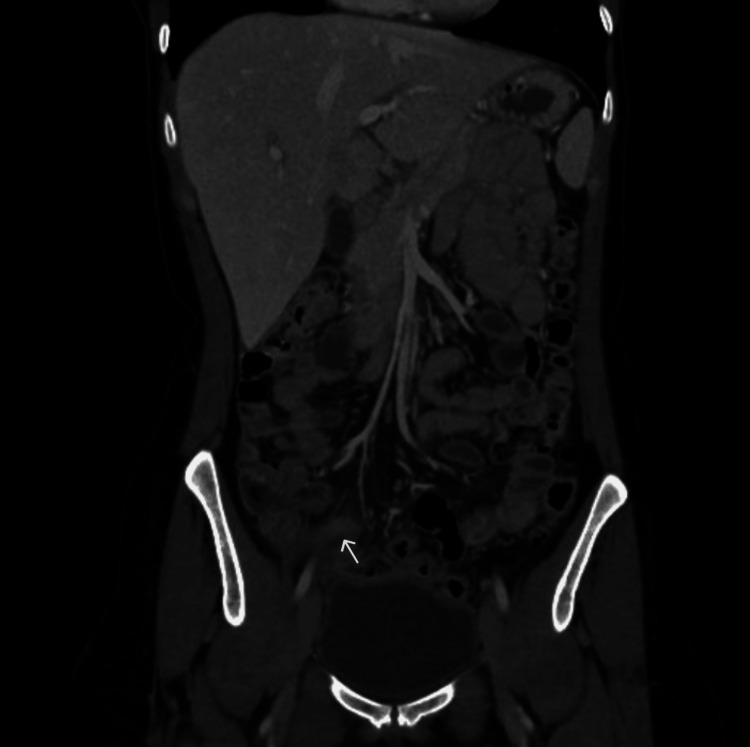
Contrast-enhanced CT of the abdomen and pelvis showing thickened and enlarged appendix (white arrow).
